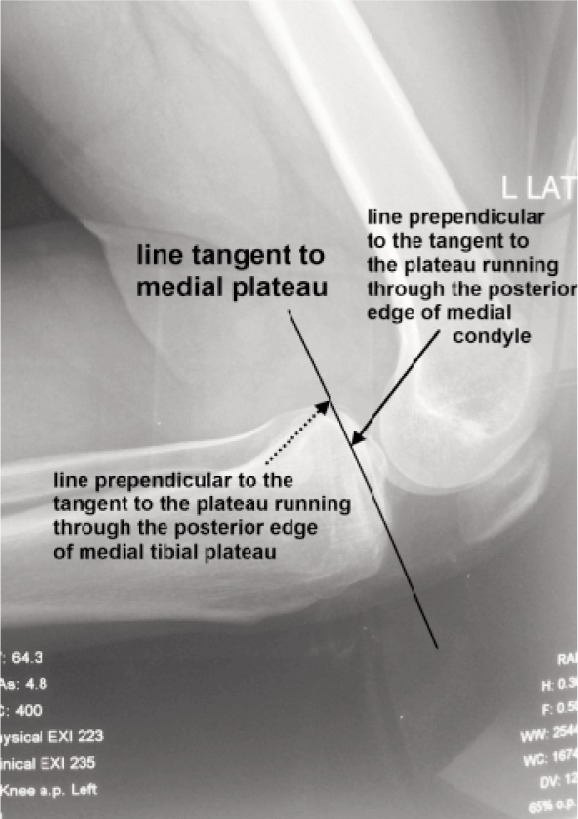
Stress radiograph to asses the posterior translation of the tibial plateau: a line is drawn tangent to the medial tibial plateau. Next a line is drawn prependicular to the tangent to the medial tibial plateau and passing through the posterior edge of medial tibial plateau. Subsequently, another line is drawn passing throught the posterior aspect of medial femoral condyle. The posterior translation is then calculated by measuring the distance between the two prependiculars drawn.
